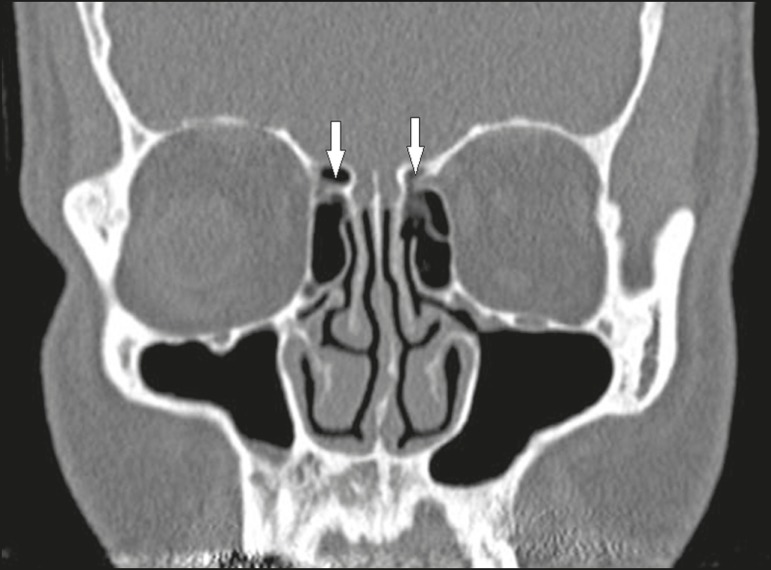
Sagittal CT slice, with bone window settings, showing the anterior
